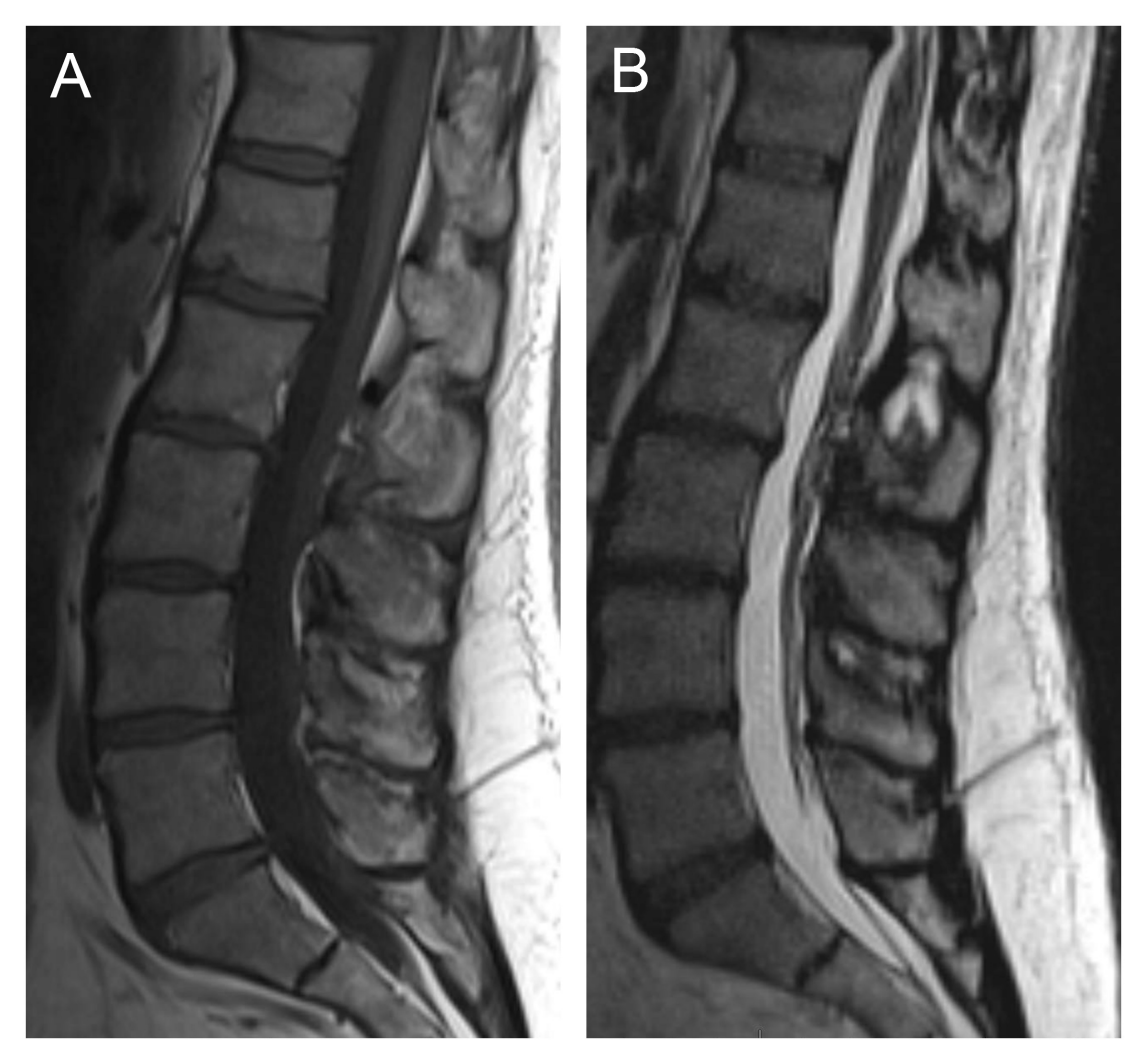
Radiographic findings on MRI T1 (A) and T2 (B) weighted imaging showing sclerotic and hypertrophic “kissing” spinous processes from L2 to L5, with mild L5-S1 facet hypertrophy
